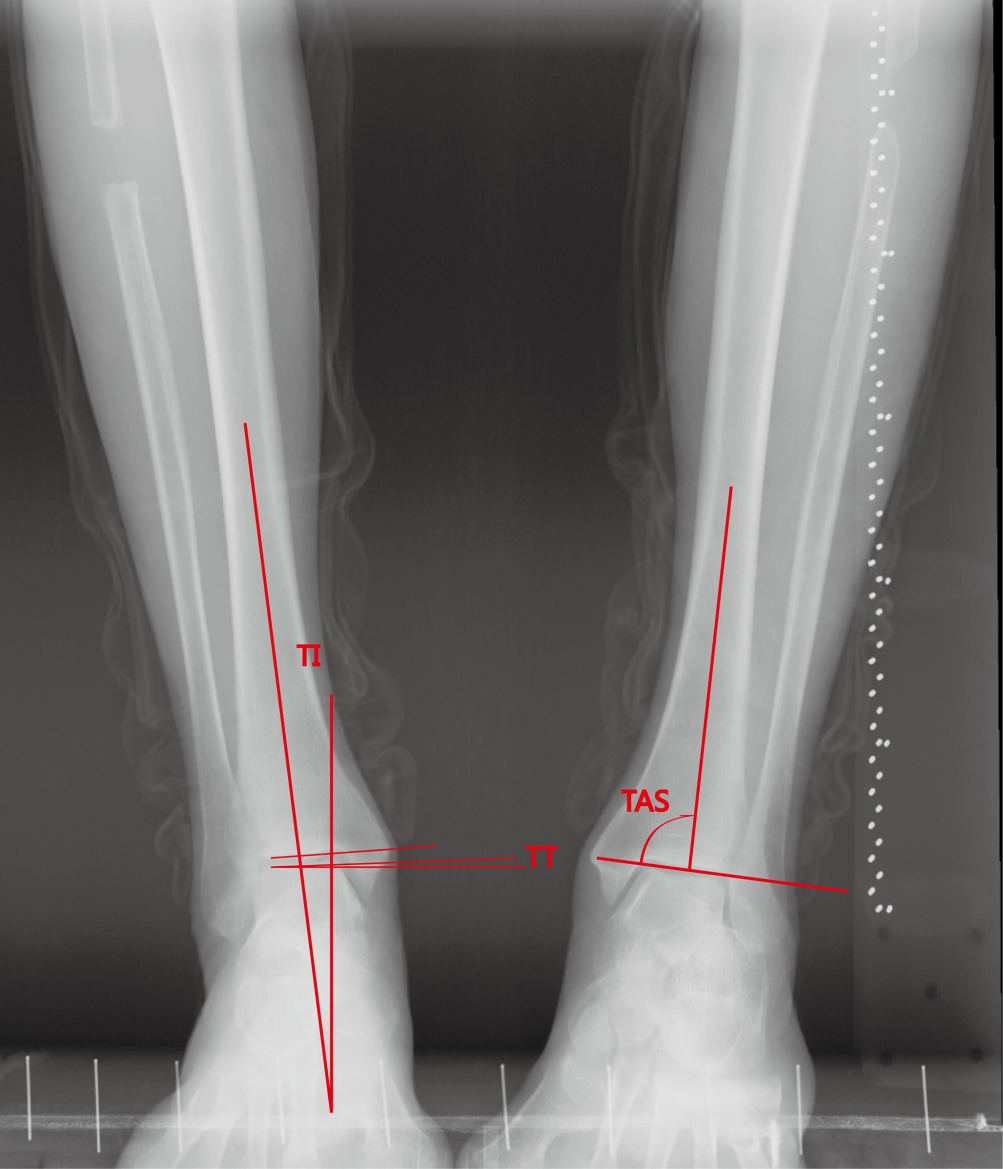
Definition of the different angles from antero-posterior radiographs. Tibial inclination (TI): acute angle between the perpendicular line and the tibial axis. Talar tilt (TT): acute angle between the tangent of the horizontal line and the superior talar surface. Distal tibial articular surface (TAS): the angle between the distal tibial joint surface and the tibial shaft.
